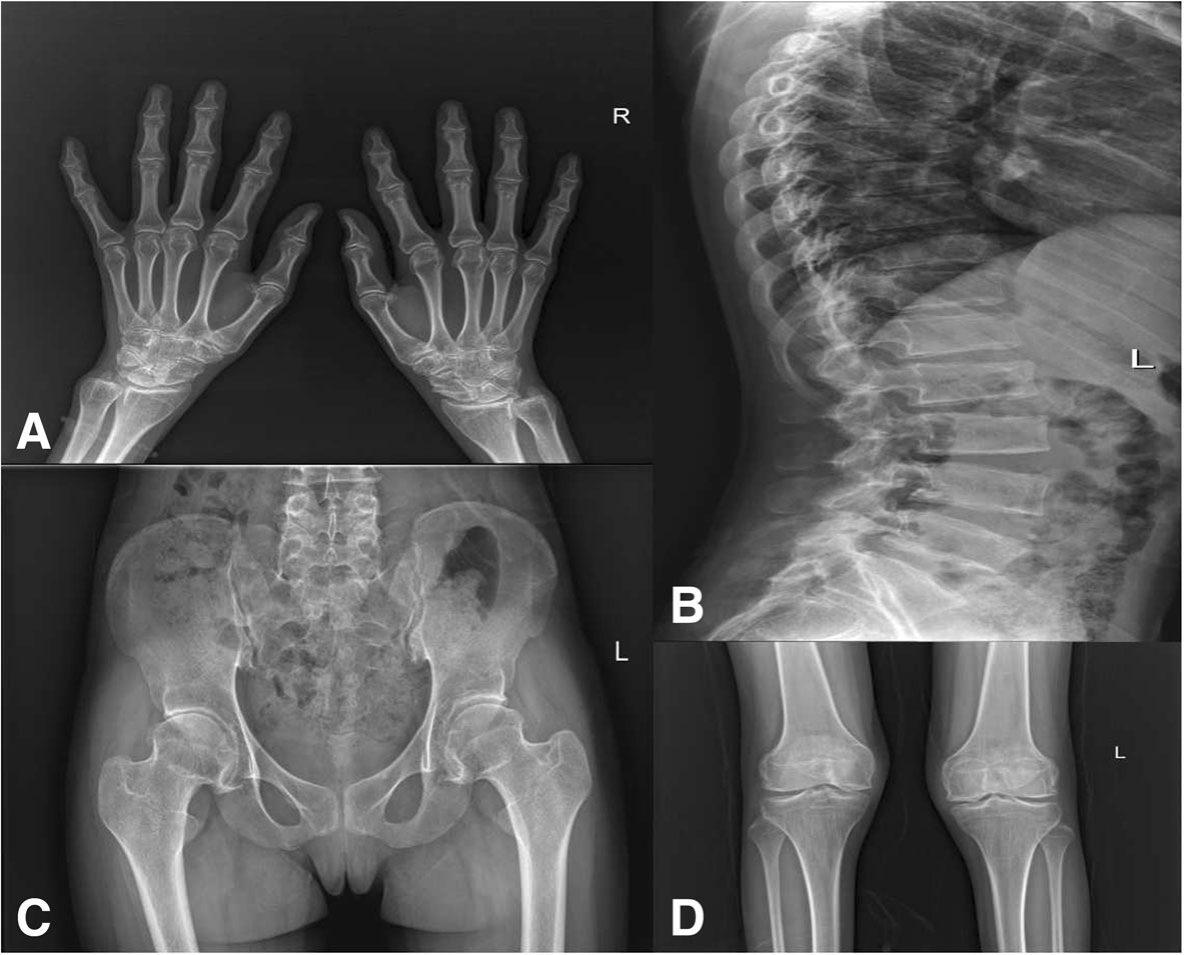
Plain films. Plain film of hands: osteoporosis (a); plain film of spine: thoracic and lumbar platyspondyly with irregular margin (b); plain film of pelvis: space narrowing of hip joint and normal sacroiliac joint (c); plain film of knee joints: joint space narrowing and rough articular surface (d)
